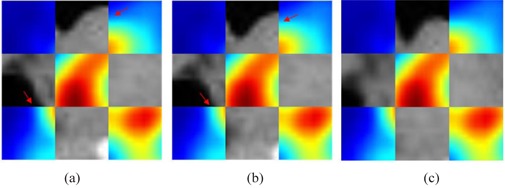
Checkerboard display of ${\;}^{18}F-\text{FDG}\;\text{PET}$ images with high metabolic lesion region and CT images: (a) before registration; (b) after global registration; (c) after GMI demons algorithm registration.
